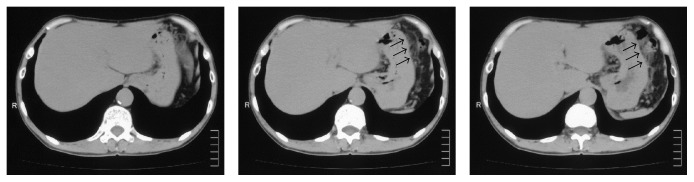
Abdominal computed tomography before treatment. Computed tomography showed fluffing surrounding the gastric body (arrows).
